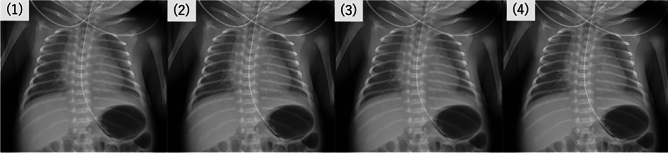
Portable chest radiographs of a 2-day-old preterm infant (34 + 3 weeks, 1,935 g). Four processing conditions are shown: (1) standard (no processing), (2) edge enhancement only, (3) noise reduction only, and (4) combined noise reduction and edge enhancement
